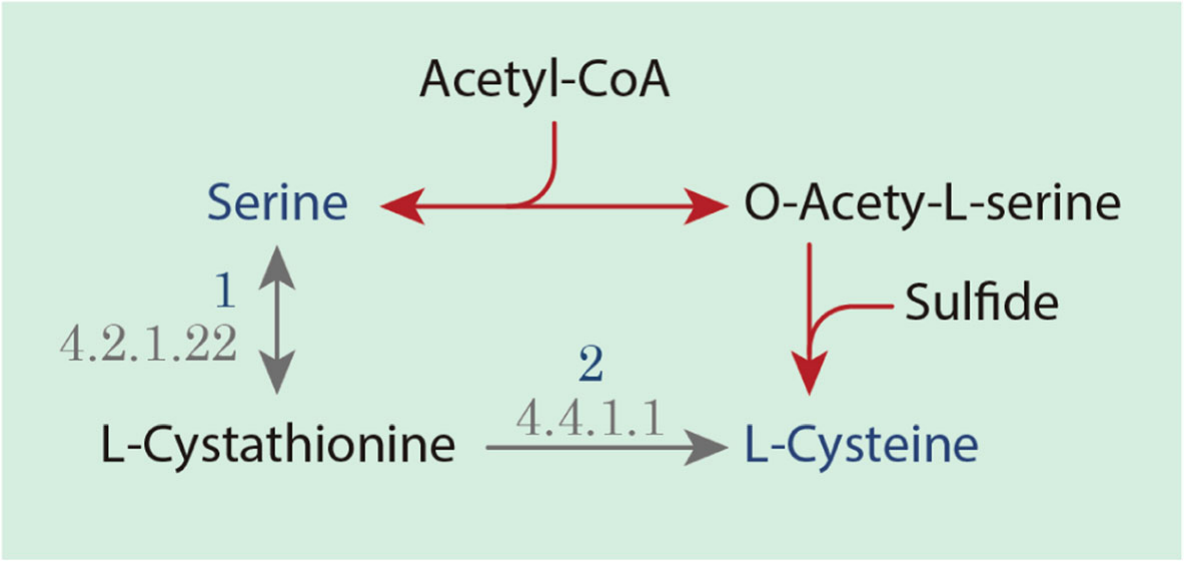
Prediction of Cysteine biosynthesis pathways according to the metabolic annotation of *P. irregulare* CBS 494.86. Detailed legend: The black arrows represent cystathionine (CT) pathway, the most probable pathway applied by *P irregulare* CBS 494.86 according to its metabolic annotation; red arrows represent the O-acetylserine (OAS) pathway
